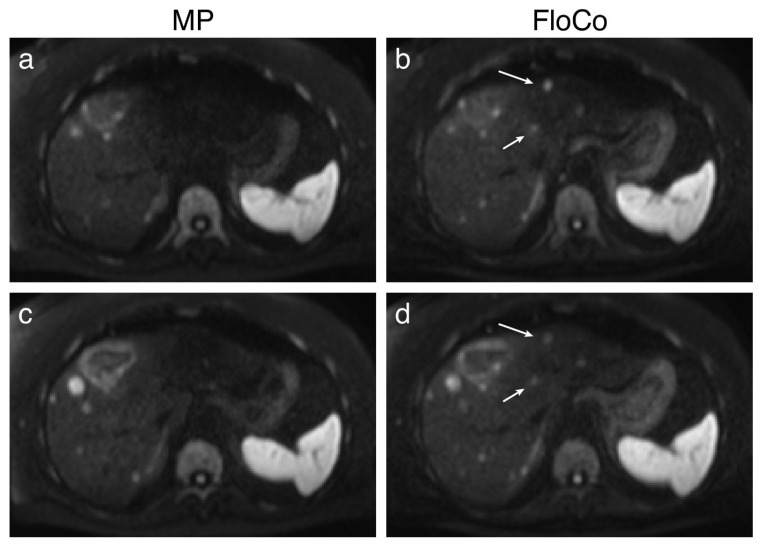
Liver diffusion-weighted MRI (b = 800 s/mm^2^) of a patient with metastatic neuroendocrine tumor. Examples of focal liver lesions (FLLs) visible only in flow-compensated (FloCo) DWI. (a,c) Monopolar (MP) DWI, two adjacent slices; (b,d) FloCo DWI, two adjacent slices. The arrows highlight two FLLs in segments II and IV that were not detectable with MP DWI. In this example, the FLLs were labeled as “only_floco”.
